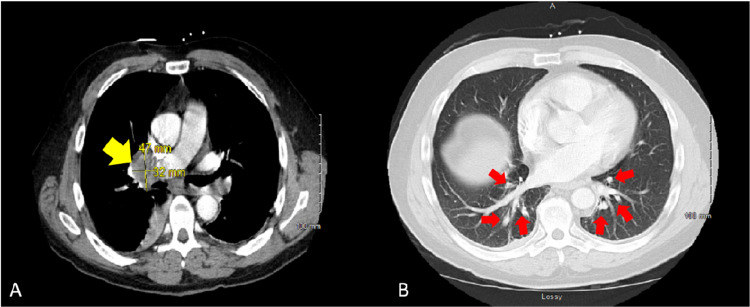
Computed tomography angiography (CTA) of the chest A) large right hilar mass measuring 47 x 32 mm (yellow arrow) in association with consolidation to the medial right lower lobe, having a compressive effect upon the second-order airway branches to the right lower lobe; B) presence of bilateral hilar lymphadenopathies (red arrows)
